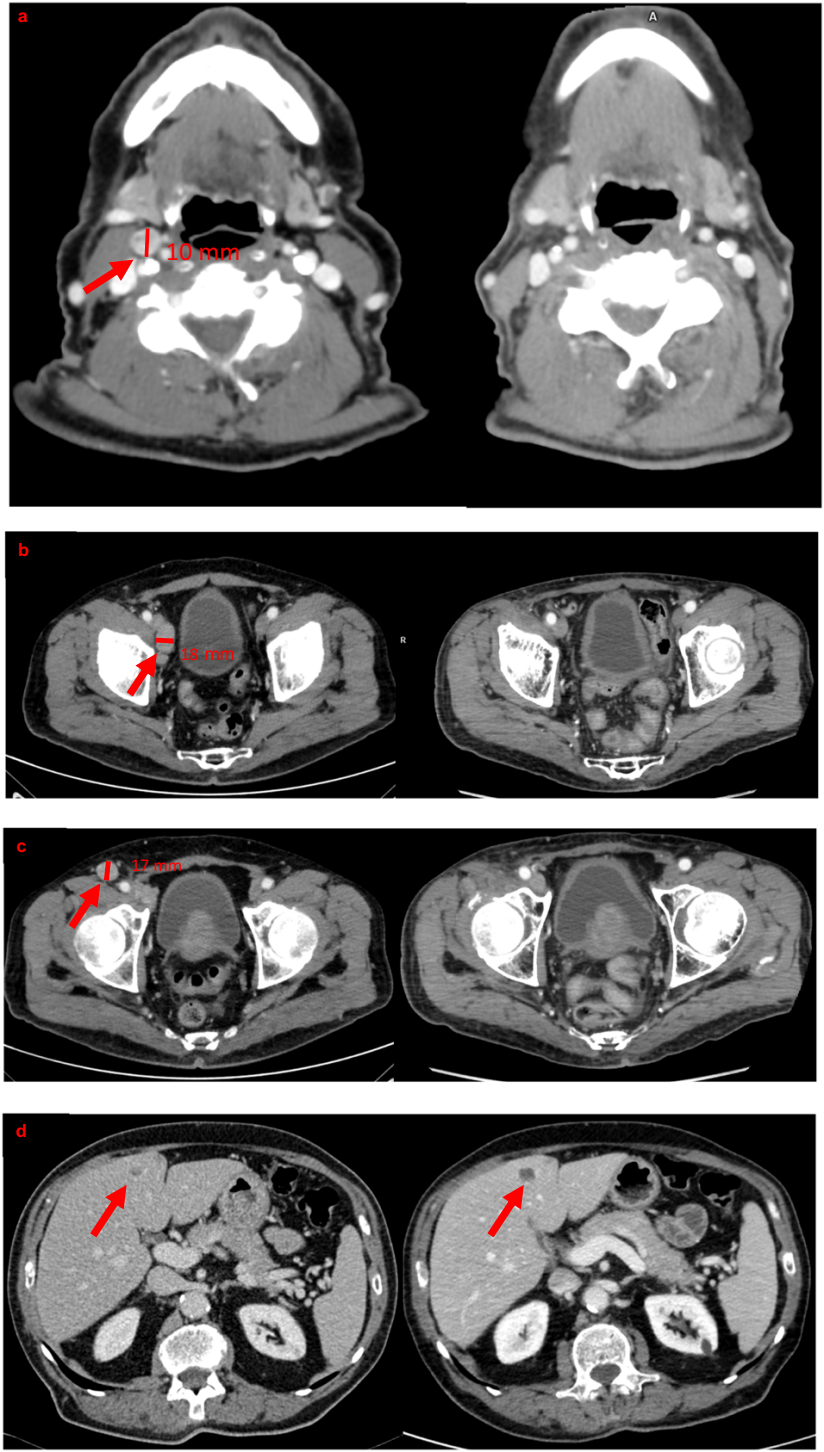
On the left baseline CT scan (before cabozantinib start); on the right CT scan of the biochemical and radiological nadir (after 22 months). a On the left CT scan showed pathological latero-cervical lymph node (11 × 10 mm); while on the right, no pathological lymph node is evident. b On the left CT scan showed right external inguinal lymph node (27 × 18 mm); while on the right, no pathological lymph node is present. c On the left CT scan showed right inguinal lymph node (19 × 17 mm); while on the right, no pathological lymph node is present. d CT scan showed pseudoprogression of hypodense hepatic lesion in the IV segment between baseline and after 22 months
